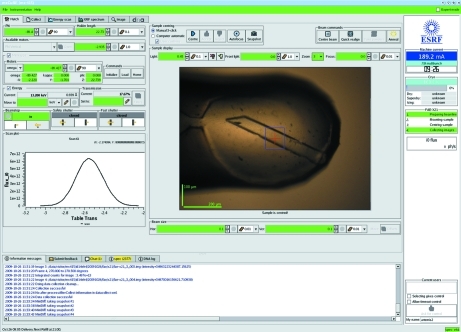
The Hutch tab of the *MxCuBE* GUI. Expert mode is accessed by clicking the check box in the top right of the GUI. The plot window shows a scan of beam intensity *versus* horizontal translation of the experiment table carried out as part of the ‘quick realign’ procedure. The image-viewing brick shows a sample after centring in the X-ray beam (blue square) using the 3-click option.
